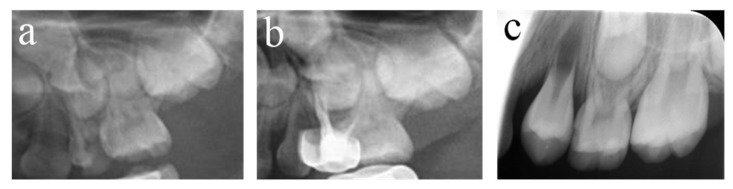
#64 received pulpectomies under DGA with a 32-month follow-up period (63-month-old male child). (a) The preoperative radiograph revealed nothing abnormal in the periapical area. (b) Radiograph taken one week postoperatively showing optimal filling. (c) Thirty-two months after the operation, #64 was lost early, and #24 erupted prematurely. Pulpectomies in #64 failed.
